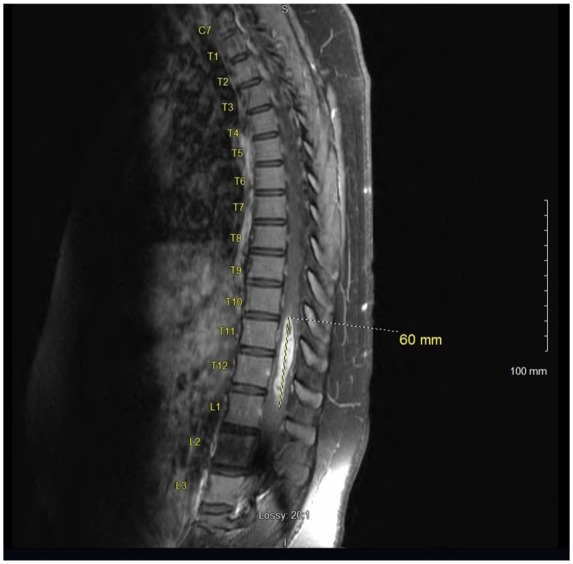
Preoperative sagittal MRI of the thoracic spine with contrast; significant for enhancing mass measuring 60 mm craniocaudal span.
